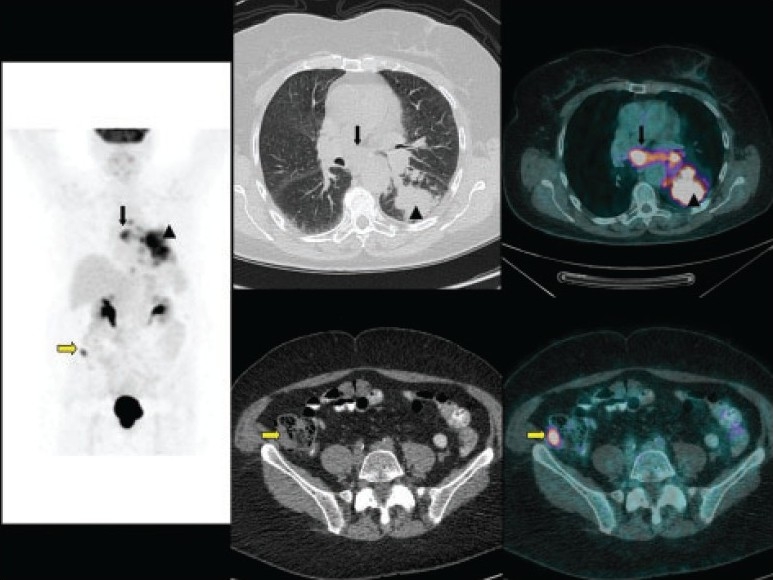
A 73-year-old woman who came for initial staging of non-small cell lung cancer. Maximum-intensity-projection (MIP) image (left panel), CT images (middle panels) and fused images (right panels) of 18F-FDG PET/CT show the primary tumor (arrow head) with mediastinal nodal metastases (black arrow). Incidental right iliac fossa small focal uptake (yellow arrow) is noted, which cross-correlated to a small soft tissue lesion in the cecum and turned out to be a synchronous primary adenocarcinoma.
